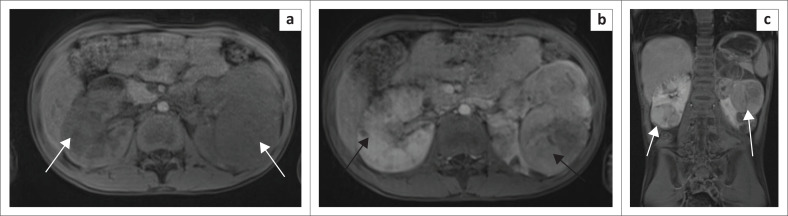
(a) T1-weighted axial abdomen demonstrates bilateral iso-intense renal mass lesions (white arrows). (b) T1-weighted axial abdomen post-contrast demonstrated enhancement of the bilateral renal mass lesions (black arrows). (c) T1-weighted coronal abdomen post-contrast demonstrates enhancement of bilateral renal mass lesions (white arrows).
